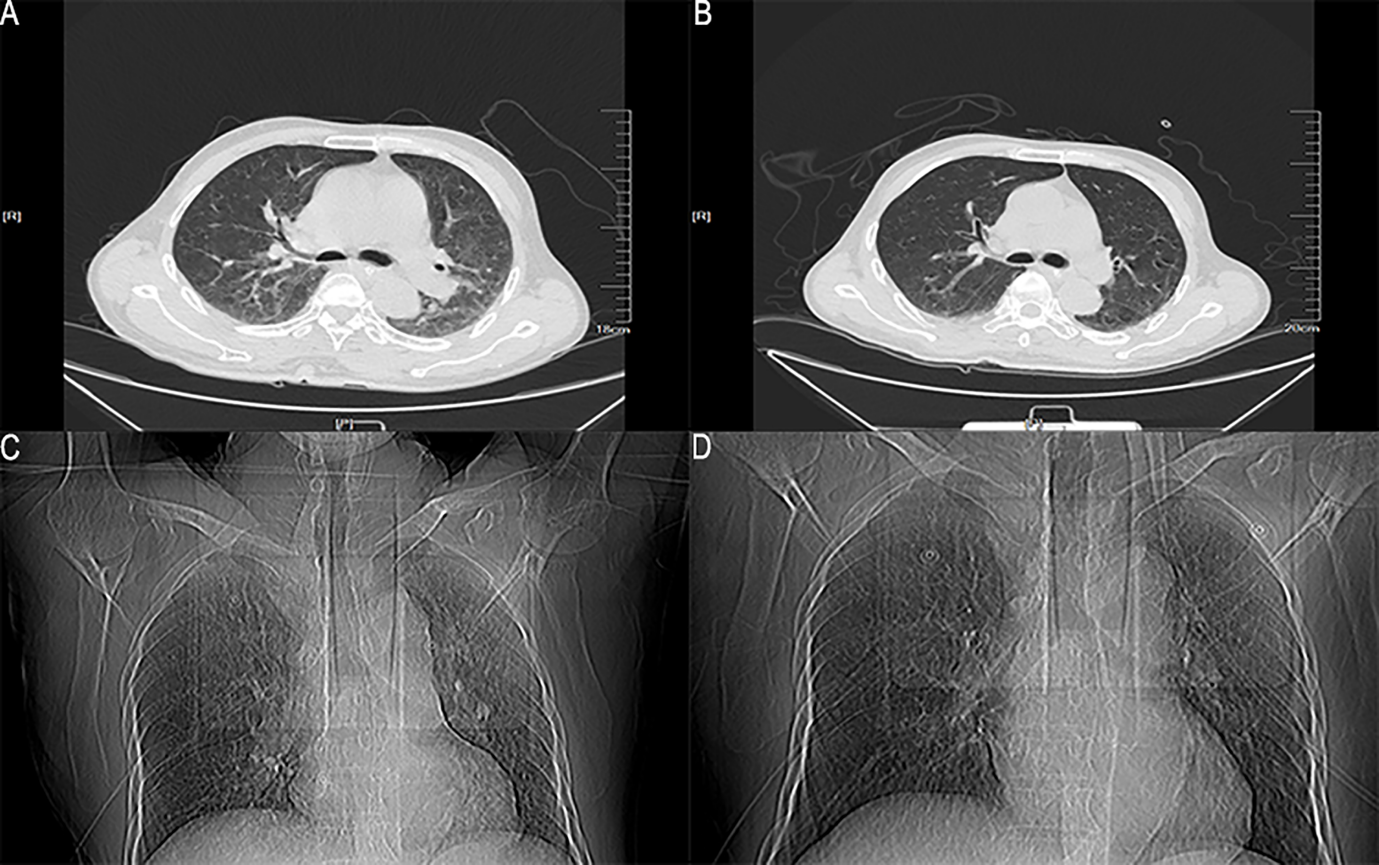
The lung window and location image of the patient’s lung on CT. (A, C) The lung CT image on the 6th day after admission; (B, D) The lung CT image on the 13th day after admission.
